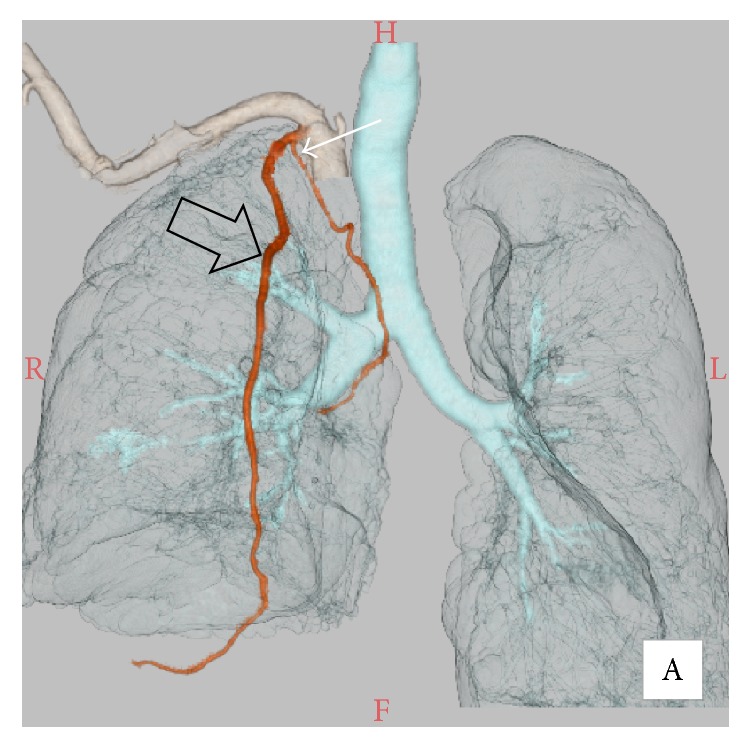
Volume rendering reconstructed image of computed tomography angiography shows a hypertrophic, aberrant right bronchial artery (white arrow) arising from the proximal portion of the right internal mammary artery (black arrow).
